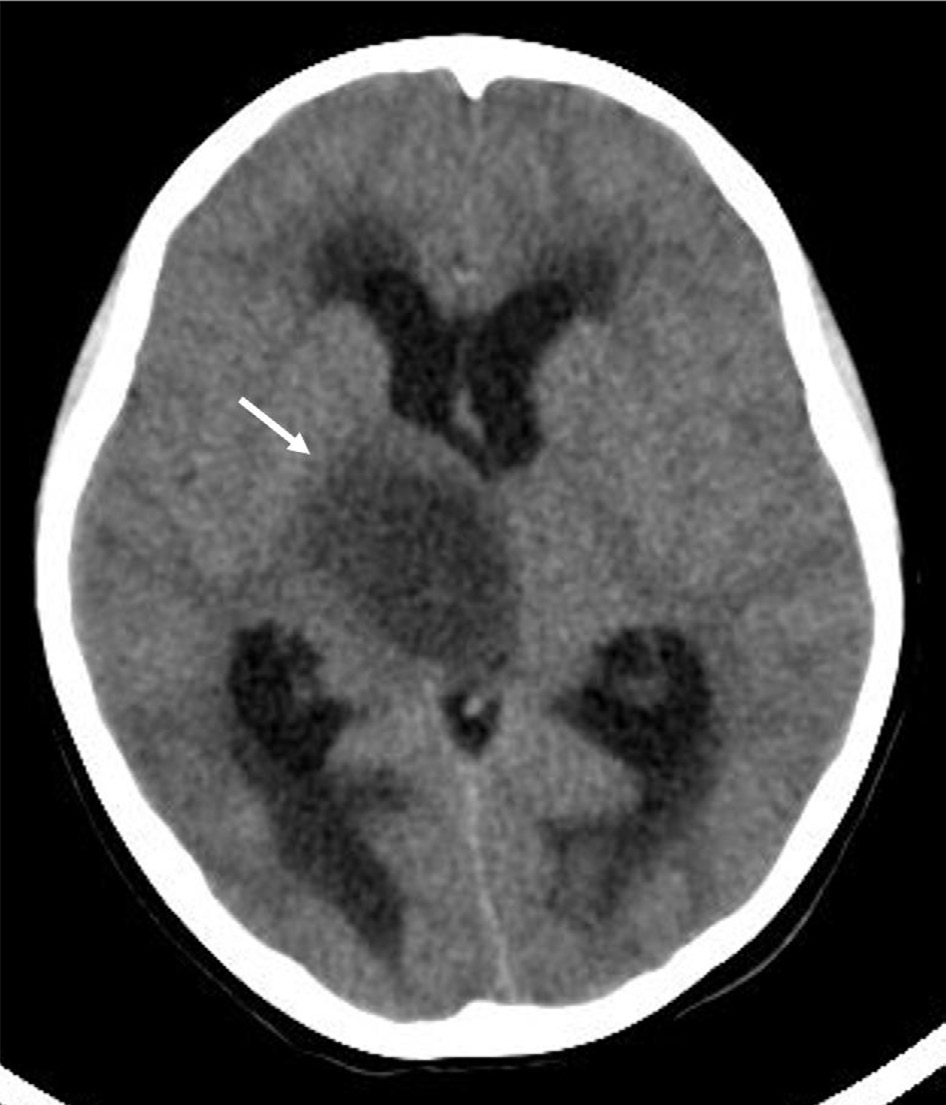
Computed tomography of the tumour (long white arrow). CT revealed an oval low-density mass in the right thalamus.
